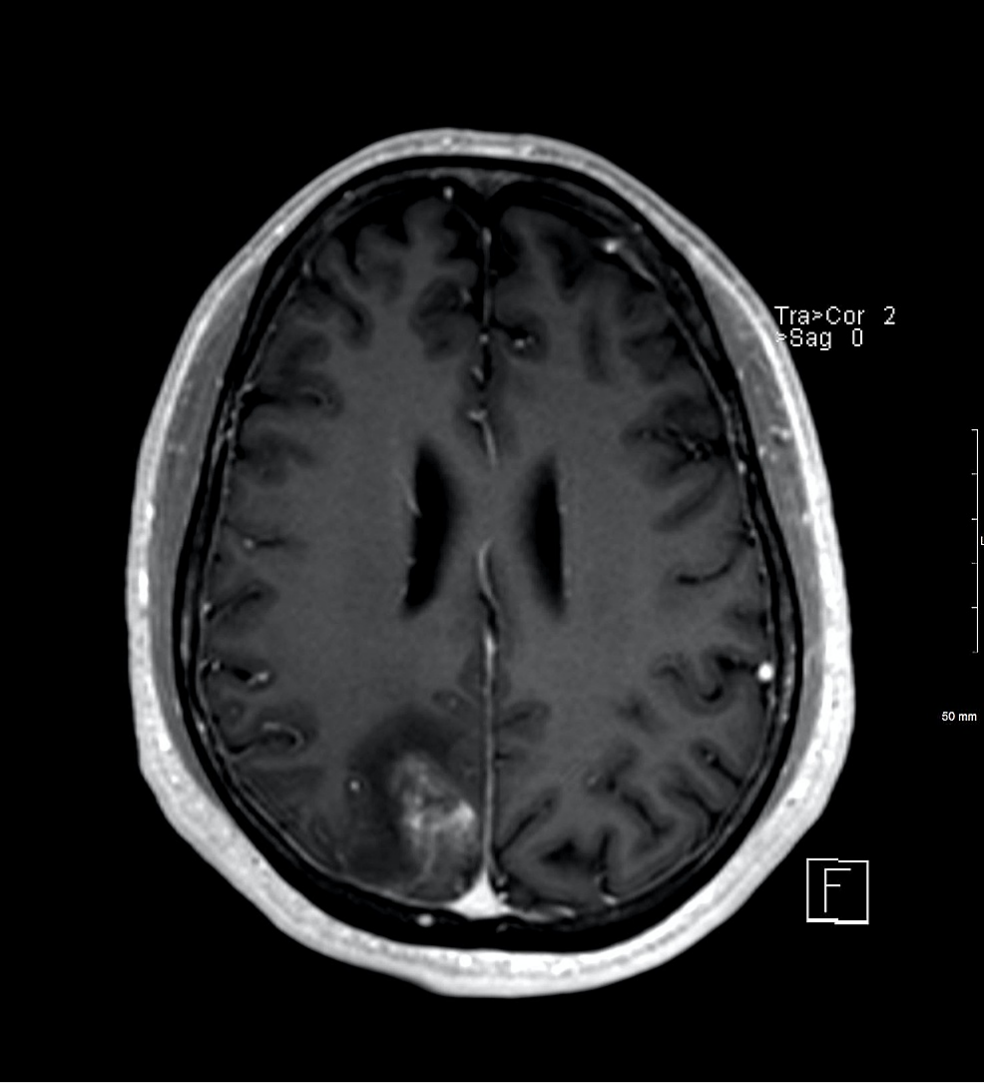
MRI brain with contrast, axial view. Hemorrhage surrounded by a small area of patchy enhancement in the right paramedian posterior lobe.
